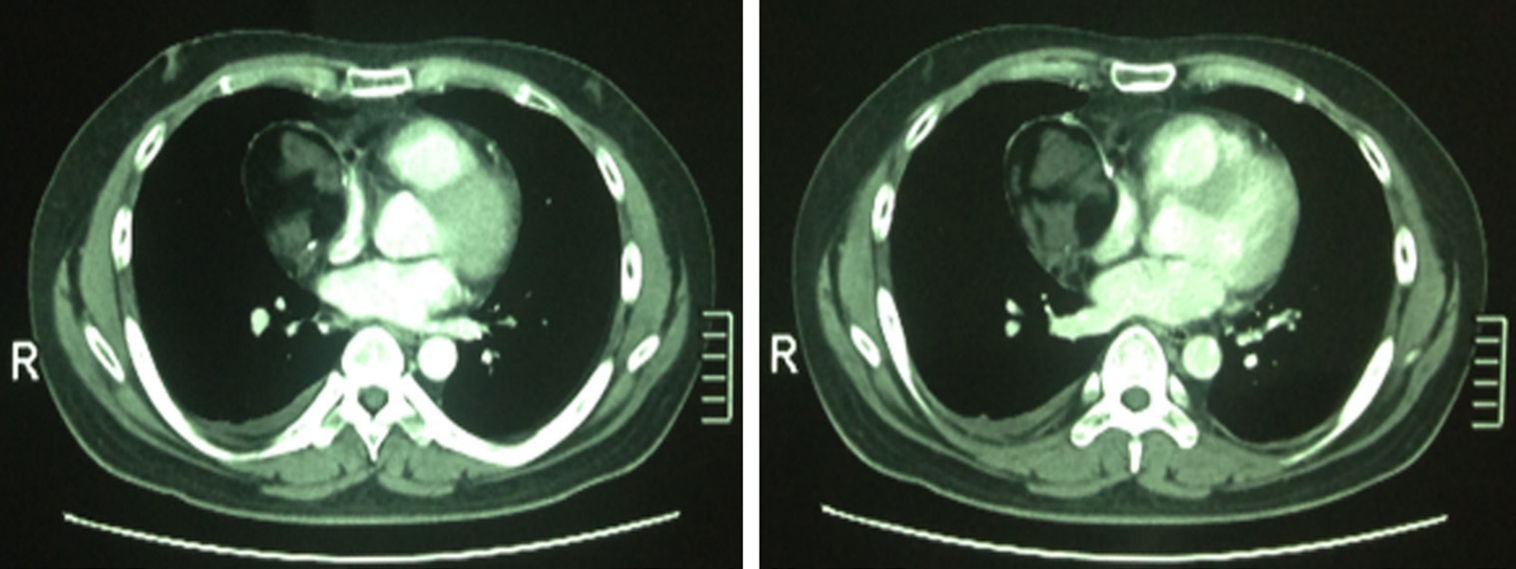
The original chest computed tomography of the patient The CT showed a well-circumscribed cystic and solid mass lesion in the right portion of the superior mediastinum measuring 6 × 5 cm with right pleural effusion.
